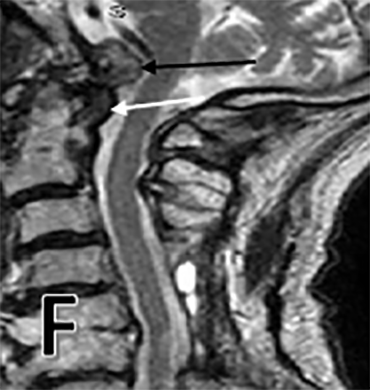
Post-operative T2 sagittal MRI showing odontoid process with reduction in pannus size (white arrow), and collapse of cystic component (black arrow).
